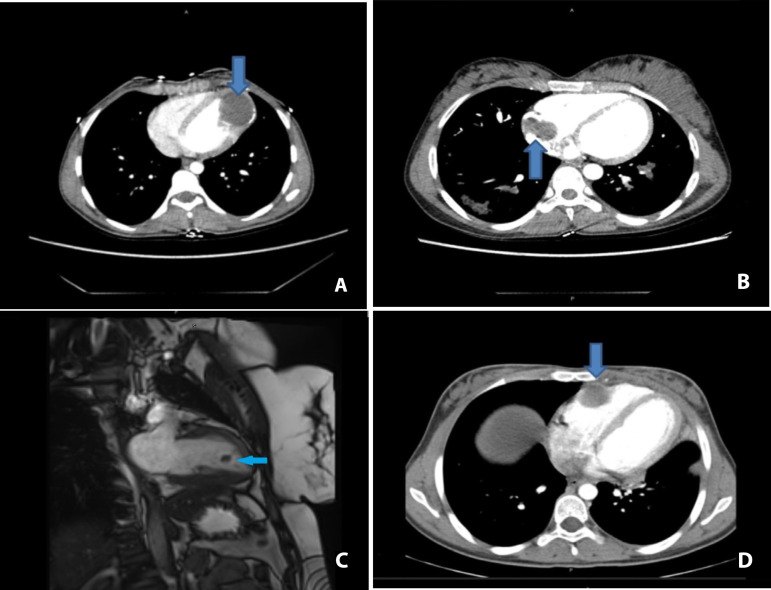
CT and MRI images of cardiac hydatid cyst.
